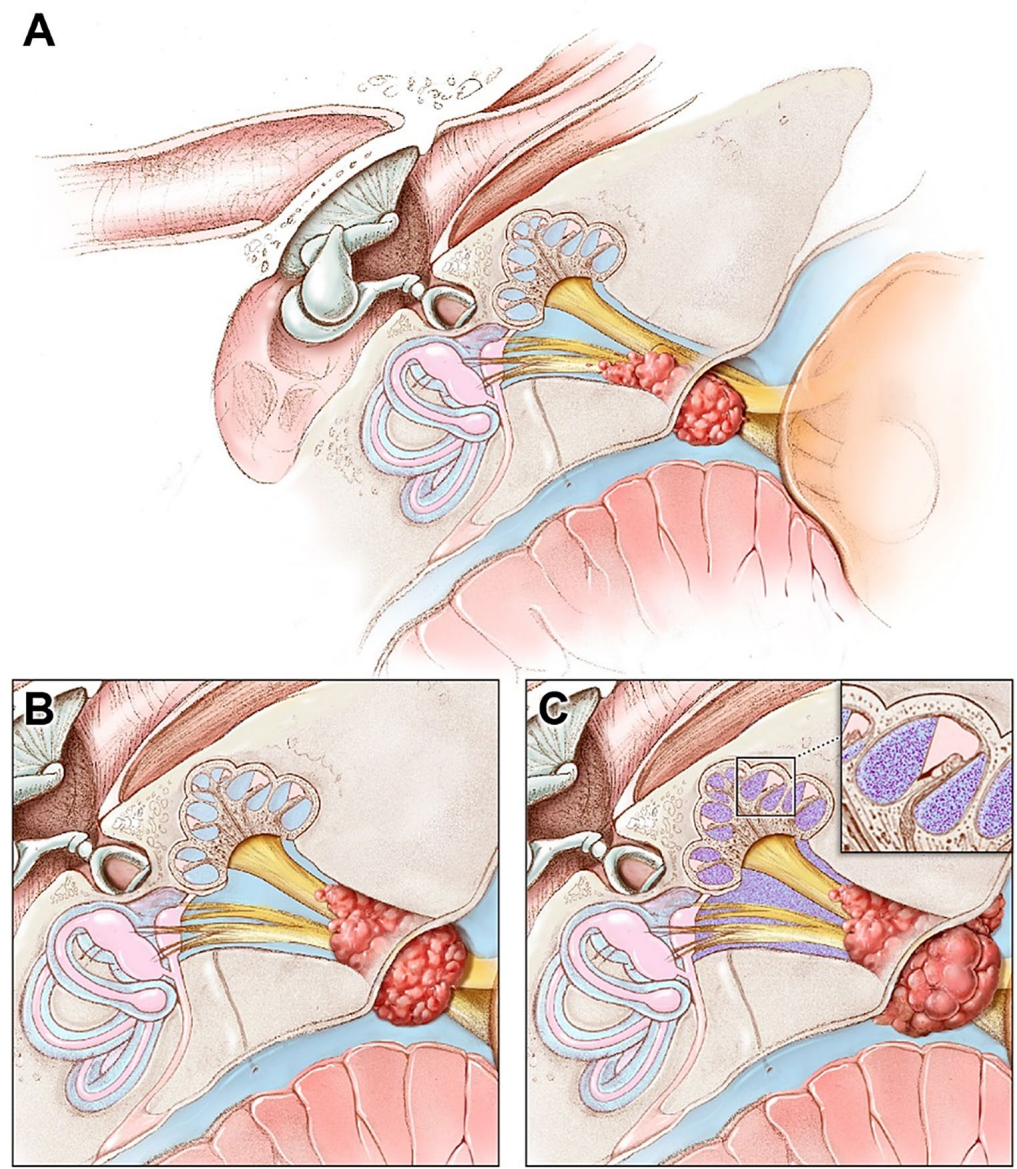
Mechanism of hearing loss in NF2. A. The VS grows by coalescing multiple tumorlets, eventually occluding the IAC. B. A key intermediary event is IAC occlusion. C. Following IAC occlusion, intralabyrinthine protein accumulates within the perilymph due to reduced outflow, which we suspect contributes to hearing loss. Intralabyrinthine protein accumulation is seen as FLAIR abnormality (C inset)
